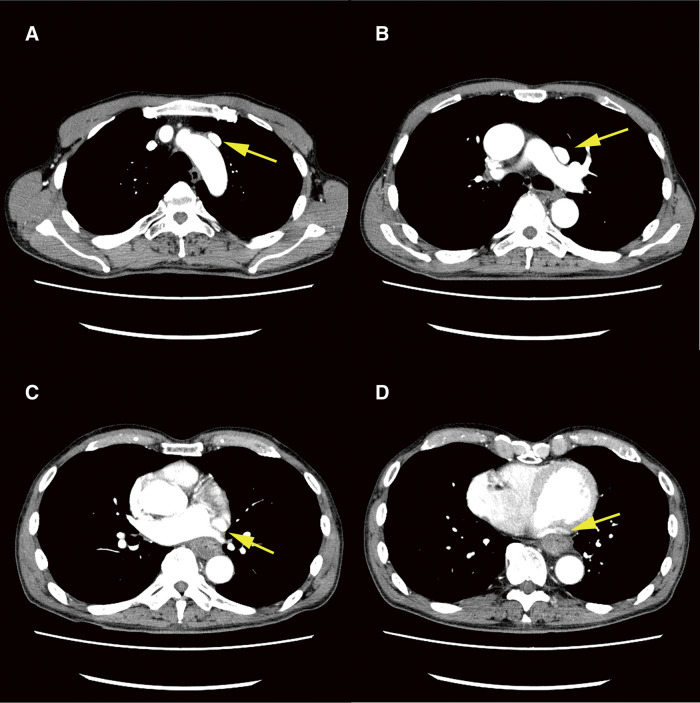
Computed tomography examination. The PLSVC runs ventral to the (A) aortic arch and the (B) left pulmonary artery. The PLSVC also communicates directly with (C) the right atrium from the left side of the left atrium (D) through the dorsal left ventricle. Yellow arrows: PLSVC. PLSVC, persistent left superior vena cava
